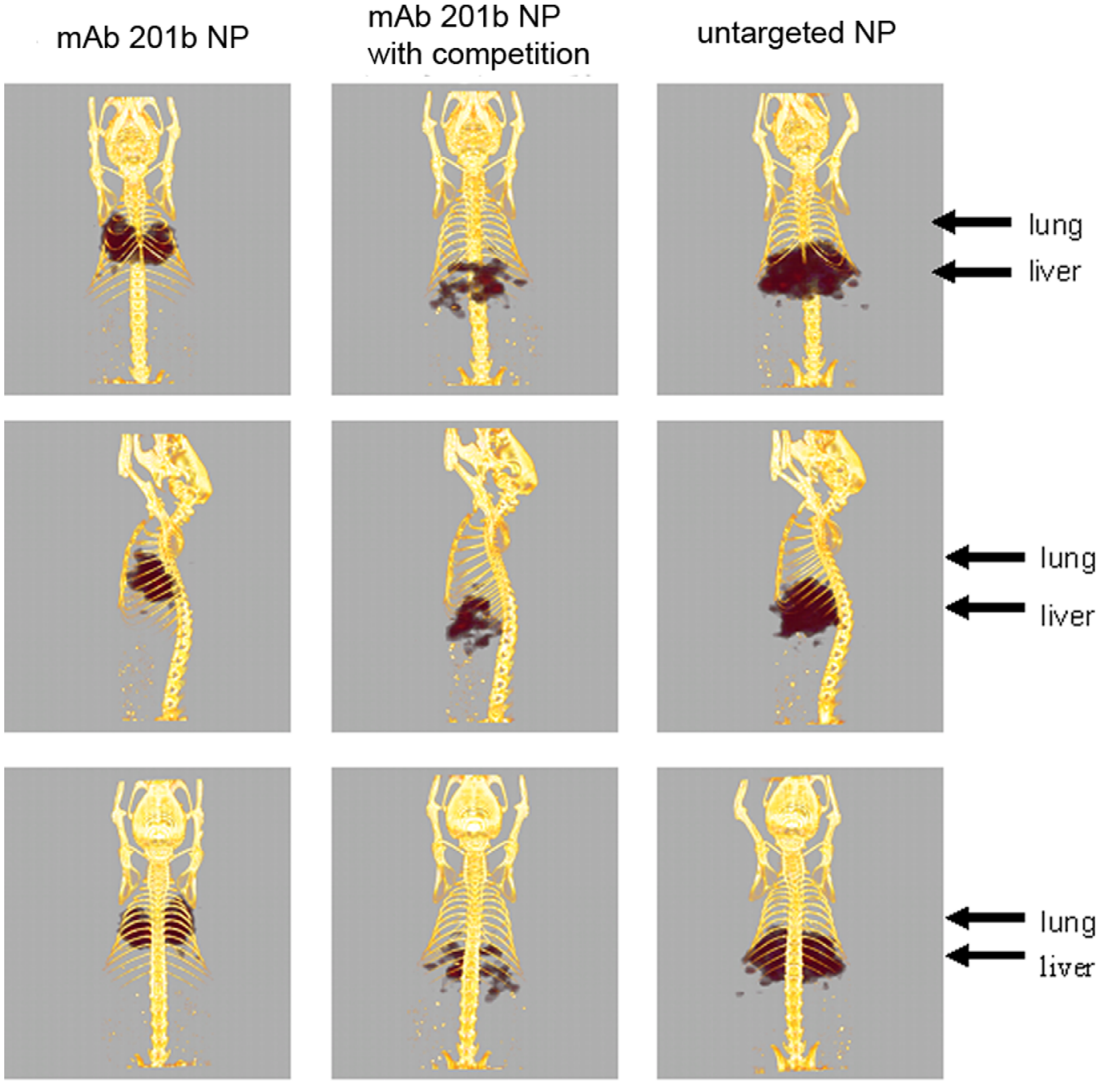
SPECT/CT images 1 hour post-injection of 80 µCi of {La_0.5_Gd_0.5_}(^225^Ac)PO_4_@GdPO_4_@Au-mAb-201b.
